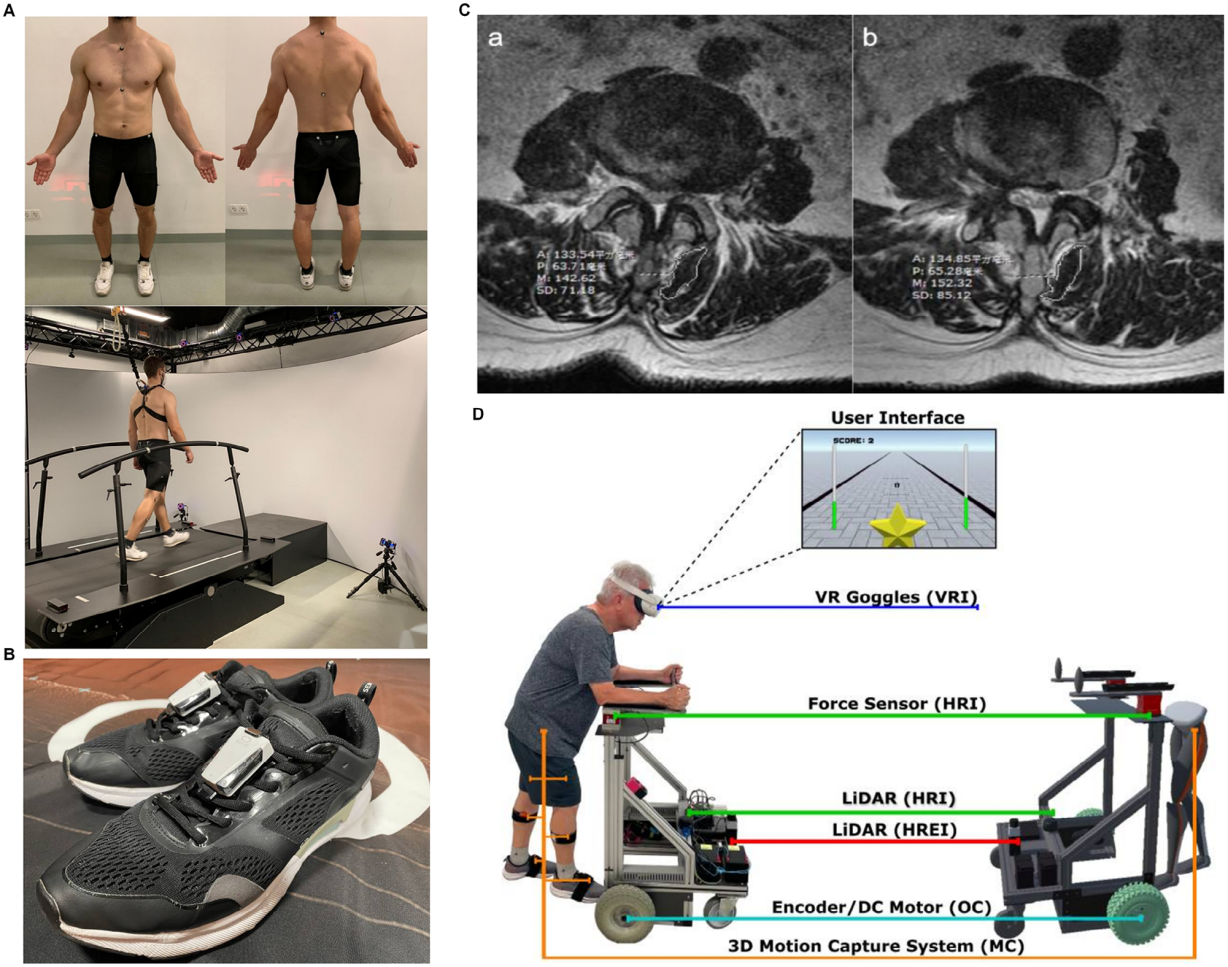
The role of AI in personalized rehabilitation programs. (A) Gait analysis dataset generation through self‐selected speed treadmill walking to determine 3D kinematic and dynamic changes. Adapted with permission [177]. Copyright © 2025, The Author(s): Stephan Oehme et al. (B) Gait data collection via wearable six‐axis sensors attached to both feet. Adapted with permission [178]. Copyright © 2025, Yoshikawa et al. (C) Axial T2‐weighted MRI analysis of lumbar muscle cross‐sectional area (CSA) using Philips MRI. Key parameters include A (CSA of the multifidus muscle in mm^2^), *P* (perimeter of the scribed area in mm), *M* (average gray value of the scribed area), and SD (standard deviation of the gray value). Adapted with permission [175]. Copyright © 2021, The Author(s): Zhen Lyu et al. (D) A participant using the immersive virtual reality scenario robot that integrates five subsystems: odometry and control (OC), human–robot‐environment interaction (HREI), human–robot interaction (HRI), motion capture (MC), and virtual reality integration (VRI). Adapted with permission [186]. Copyright © 2024, by the authors: Matheus Loureiro et al.
